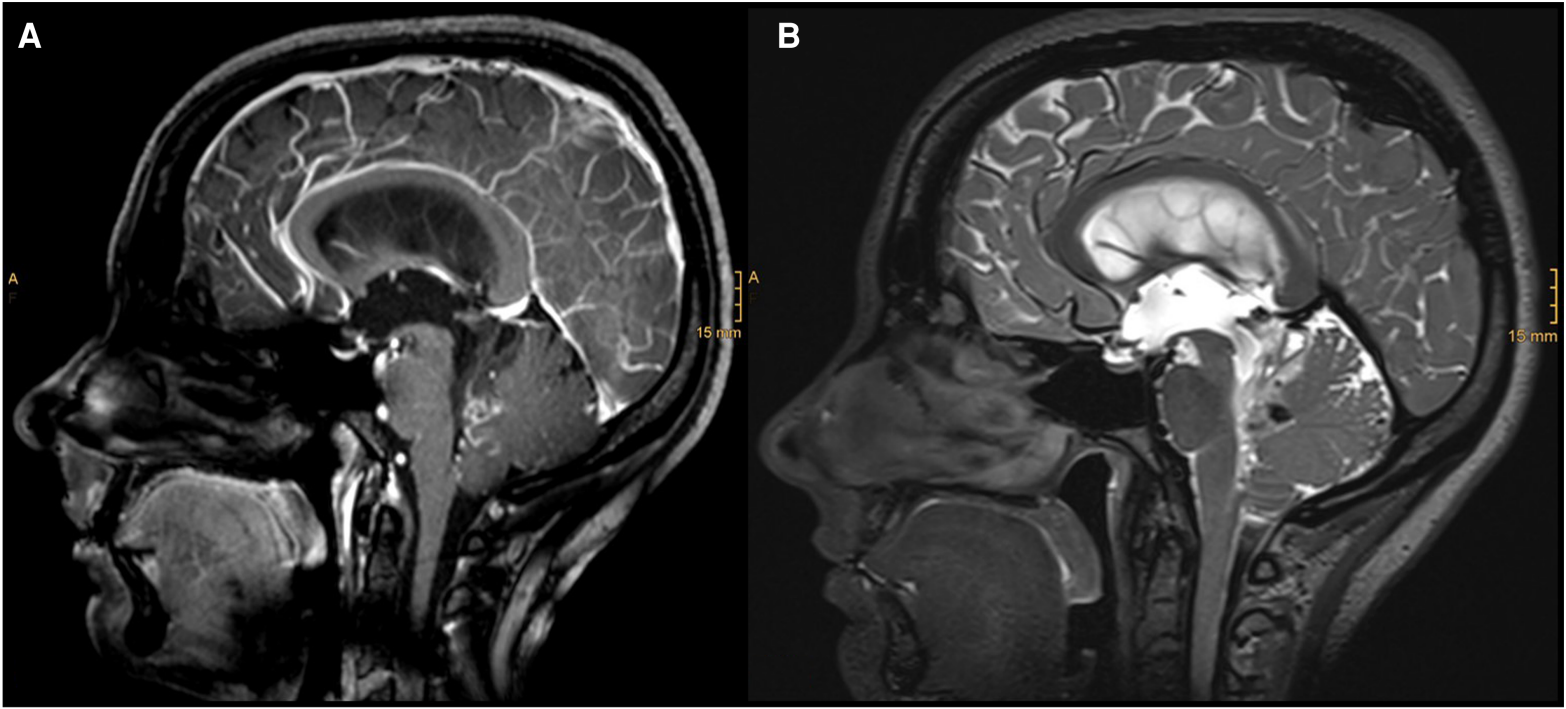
Diagnostic MRI study documenting a partially contrasted lesion of the IV ventricle with cystic components and infiltration of the vermis: (A) sagittal T1 with contrast; (B) sagittal T2.
